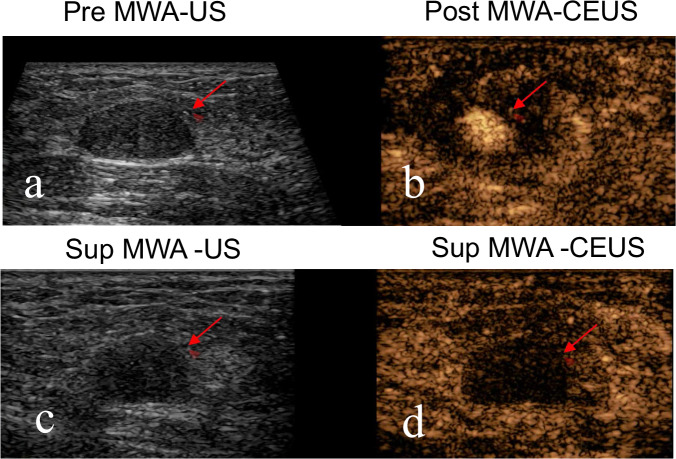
Contrast-enhanced ultrasound assessment before and after supplementary microwave ablation for residual viable tissue. a Pre-ablation ultrasound demonstrated a heterogeneous hypoechoic benign breast lesion. b Post-ablation CEUS on postoperative day 1 demonstrated residual peripheral and intralesional enhancement suggestive of viable residual tissue, prompting supplementary ablation. c Ultrasound after supplementary ablation demonstrated a heterogeneous hyperechoic ablation zone. d Repeat CEUS after supplementary ablation confirmed complete non-enhancement of the treated lesion. MWA, microwave ablation; US, ultrasound; CEUS, contrast-enhanced ultrasound; Sup, supplementary
